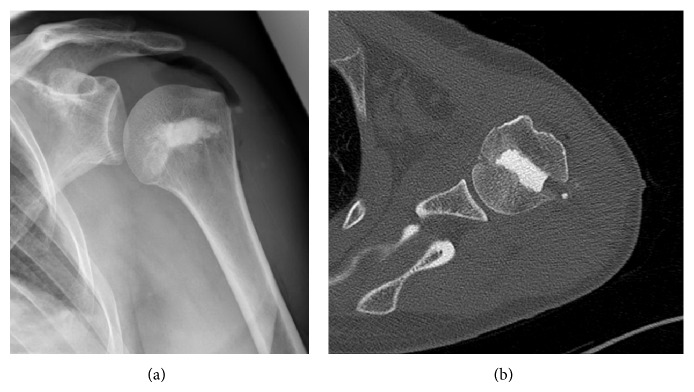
X-ray (a) and CT (b) two days after index procedure showing an intact glenohumeral articulation and a restored humeral head.
